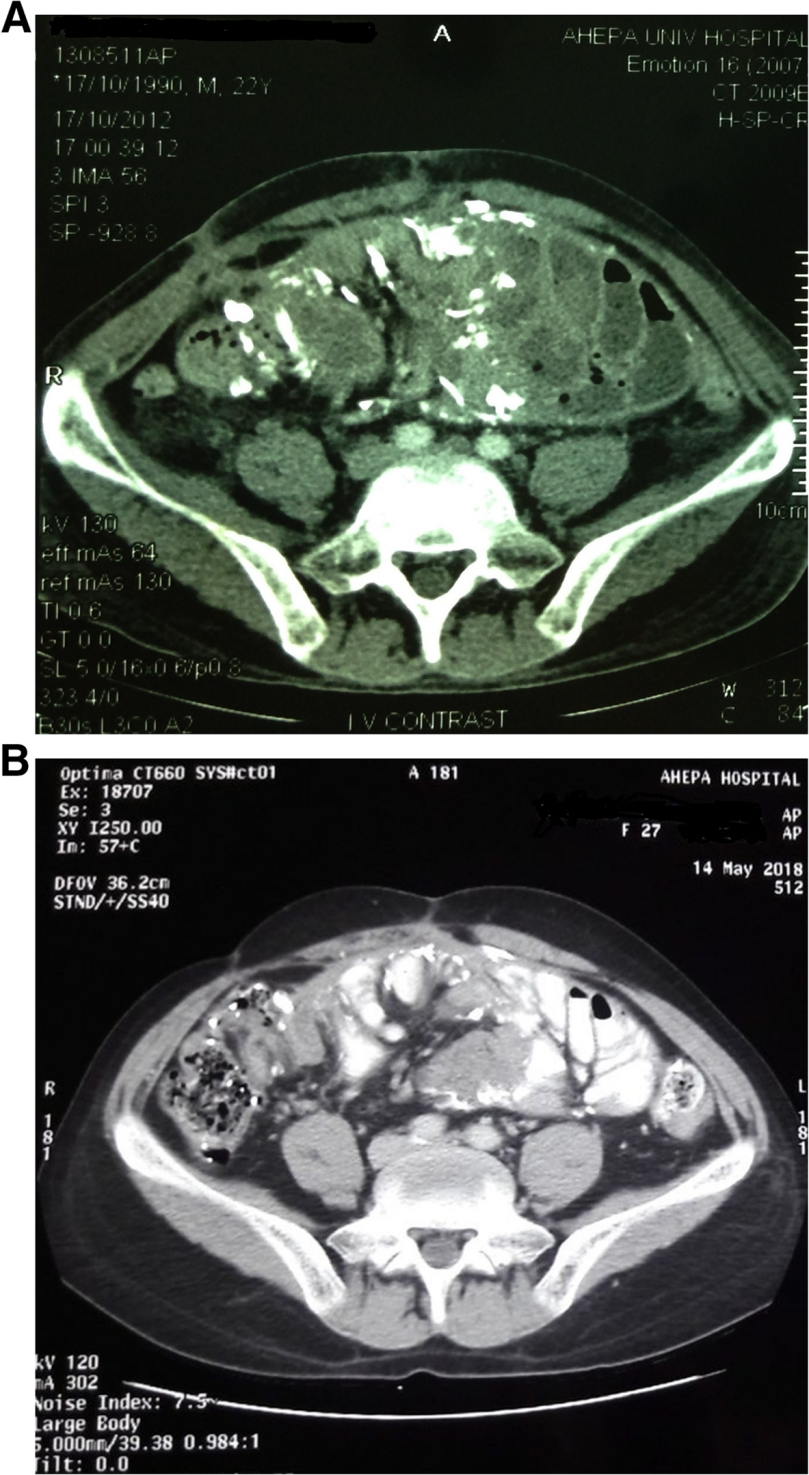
a Abdominal CT scan after the discontinuation of steroids (Oct 2012) showing a similar radiological picture in comparison with earlier CT scans; b abdominal CT scan (May 2018) showing minimal improvement of the radiological picture over a 10-year-long follow-up. The high density in (b) is due to oral intake of a water-soluble contrast agent for the abdominal CT scan evaluation
